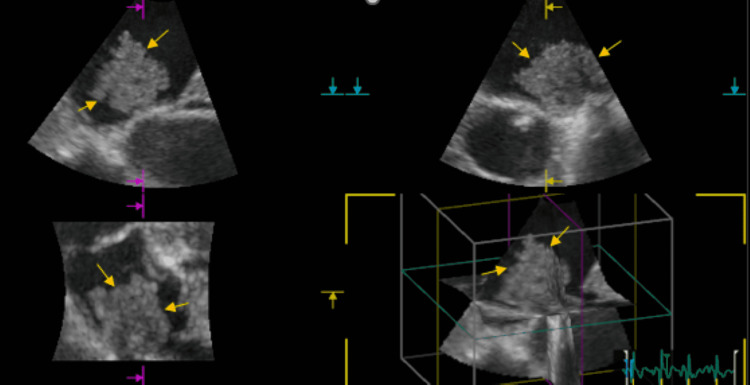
3D echocardiography showing a 2 cm X 2.1 cm mobile mass seen in the left atrium
